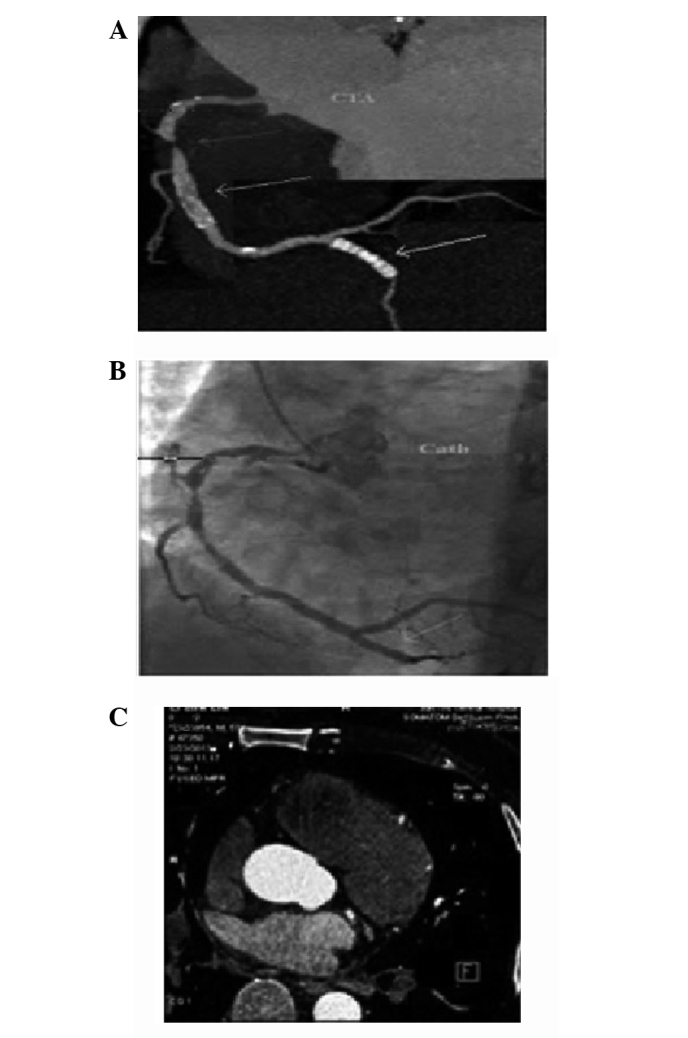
(A) Flash DS-CT, the nearly whole block of the proximal RCA. (B) CAG, the nearly whole block of the nearly RCA. (C) Flash DS-CT, defect of myocardial perfusion at the inferior wall. DS-CT, dual-source-CT; CAG, coronary angiography, RCA, right coronary artery.
